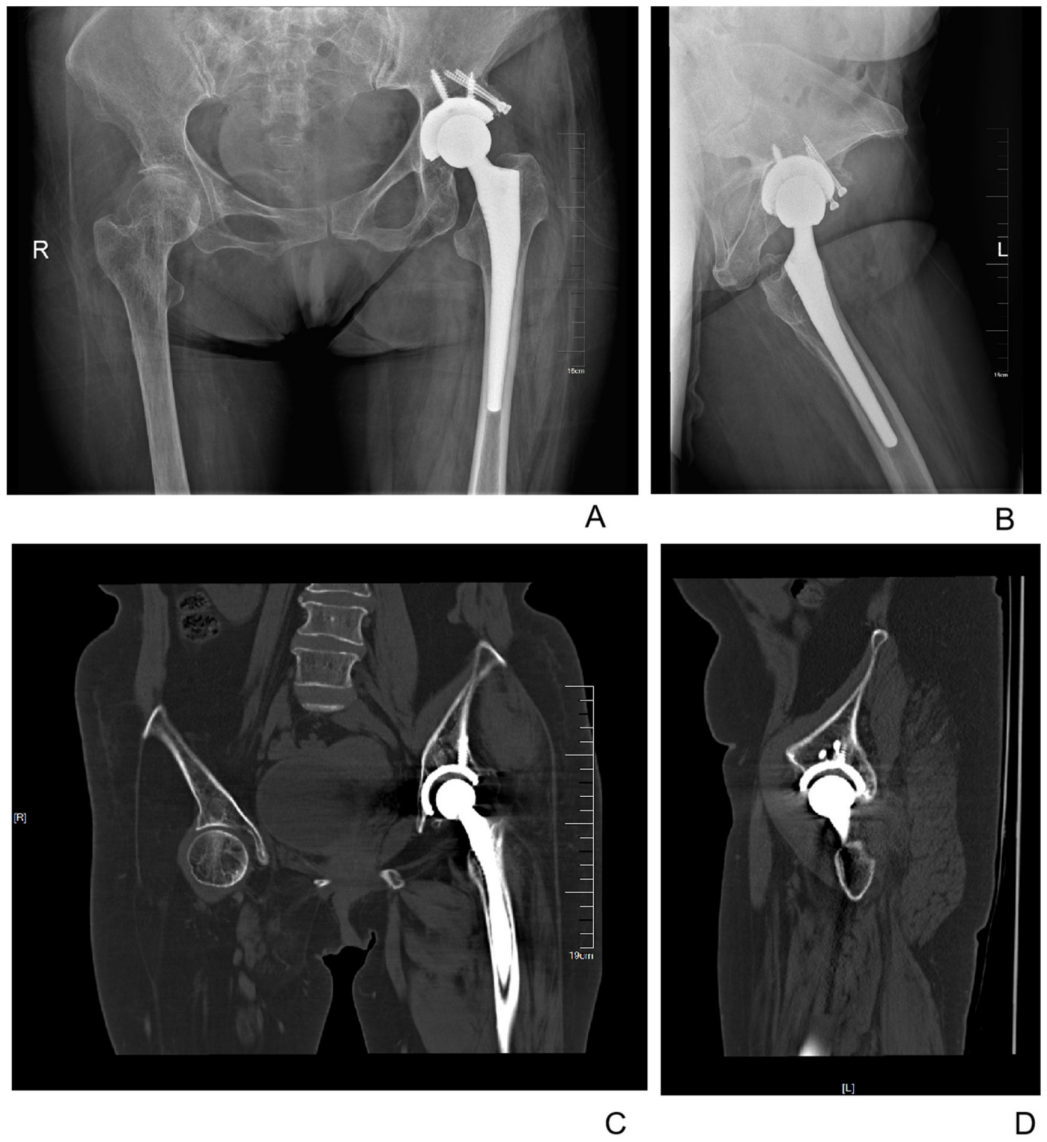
Postoperative X-ray and CT images of the female patient at 1 year after surgery. (A) Anteroposterior radiograph of the hips. (B) Lateral radiograph of the left hip. (C) Anteroposterior CT image of the hips. (D) Lateral CT image of the left hip.
